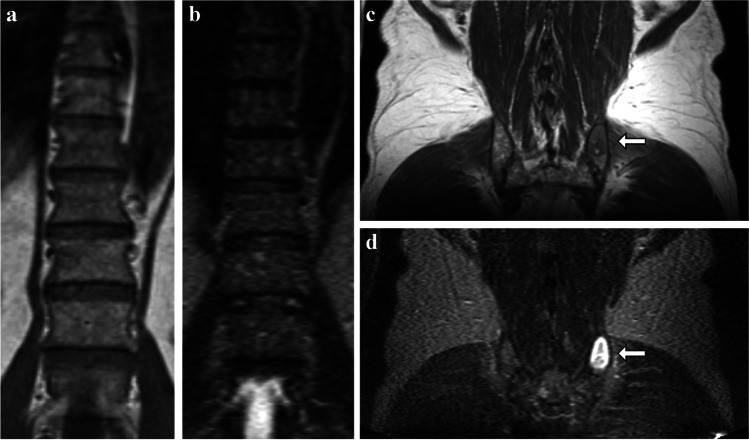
a–d Case 1. A 62-year-old male with smoldering multiple myeloma after coincidental biopsy of a large focal lesion. Coronal T1tse (a, c) and STIR (b, d) MR images show low to intermediate diffuse infiltration in spine (a, b) and pelvis (c, d). However, a biopsy-based PCI of 90% was reported for the bone marrow. This wb-MRI reveals that a focal lesion at the left posterior iliac bone was coincidentally hit in the biopsy (c, d, white arrows)
